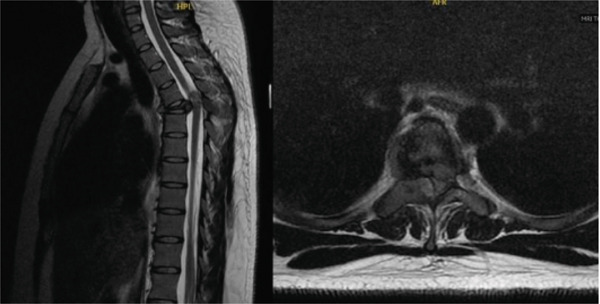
MRI imaging showing (a) axial T2 at the level of T5 and (b) sagittal T2 at the level of T5.
